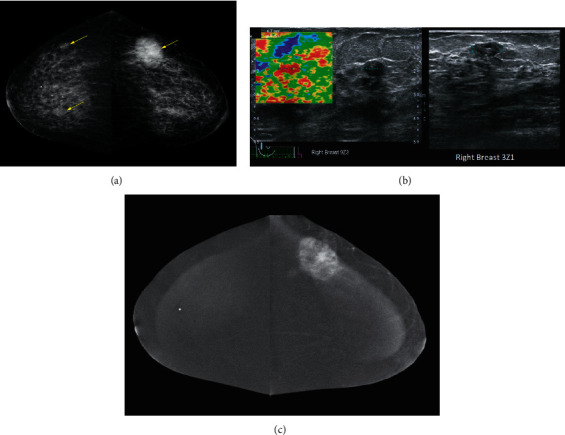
A 60-year-old woman with a palpable left breast lump. (a) Craniocaudal views of both breasts showed masses in the outer and inner halves of the right breast and a large mass associated with suspicious calcifications in the outer half of the left breast (yellow arrows). The left breast mass had yielded invasive ductal carcinoma on biopsy. (b) Selected US images of the right breast showed a stiff, solid nodule in the 0900 position of the right breast and another hypoechoic nodule with a cystic component with a mildly angular margin in the 0300 position of the right breast, corresponding to the masses seen on mammogram. In the context of biopsy proven contralateral cancer, they were initially categorized as BI-RADS 4A. (c) Subtracted CESM image in the craniocaudal view showed that both lesions in the right breast did not enhance. Note the left breast cancer showed intense enhancement. US guided biopsy of both lesions in the right breast yielded fibroadenomas. With the absence of enhancement, both of the lesions in the right breast, which were initially categorized as BI-RADS 4A, could have been downgraded to BI-RADS 3. US = ultrasound, BI-RADS = breast imaging reporting and data system and CESM = contrast-enhanced spectral mammography.
